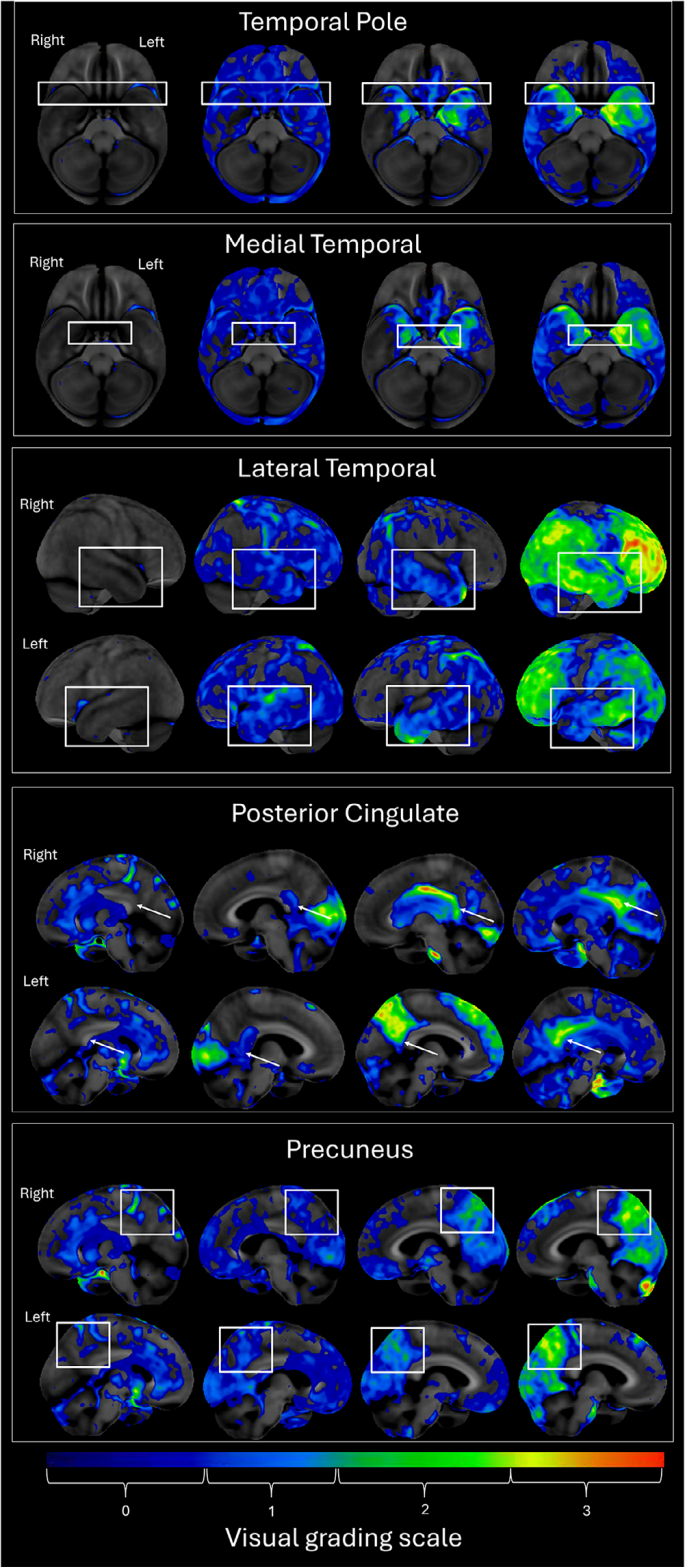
Visual grading scale for FDG‐PET hypometabolism. FDG‐PET images were processed using CortexID Suite and normalized to pons. Color overlays reflect *z*‐score deviations from age‐matched normative database. Regions were visually graded on a 0 to 3 scale based on hypometabolism severity. Colors represent *z‐*scores, not direct SUVR values. FDG‐PET, [^18^F]fluorodeoxyglucose positron emission tomography; SUVR, standard uptake value ratio.
